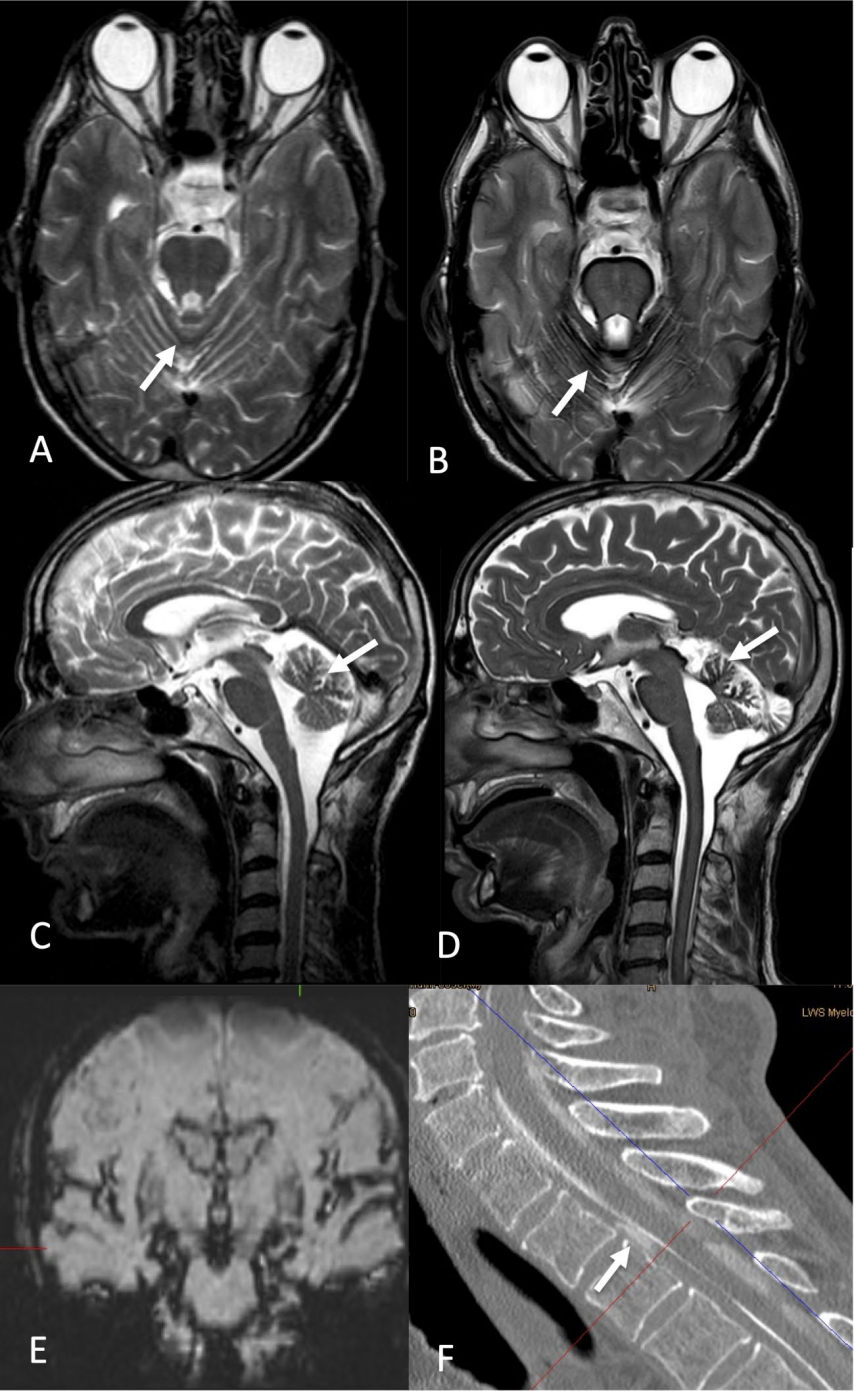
A 61-year-old man complained of vertigo progressive over some years. He could not walk stairs, had hearing difficulties, depression, and was unable to work. Initial MRI 2 years after onset of orthostatic headaches was considered normal but shows subtle infratentorial hemosiderosis (A, C: arrow). Six years later, hemosiderosis and cerebellar atrophy had progressed (B, D: arrow). A coronal SWI sequence shows the full extent of hemosiderosis (E). Work-up with dynamic DSM and CTM disclosed a ventral leak at T2/3 (F: arrow)
